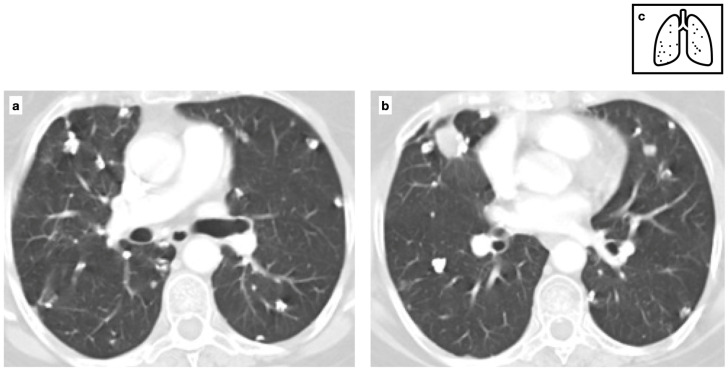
CT demonstrates diffuse calcified nodules in a patient with osteosarcoma (a,b) (Courtesy of M. Mereu). Bone formation and ossification in osteosarcoma and chondrosarcoma can result in calcified metastatic nodules of the lung. Pattern of calcification (c).
